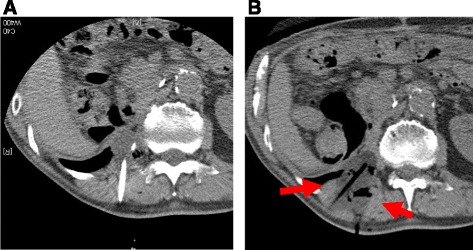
a, b. Multiple synergistic probes were inserted into the tumor to ensure complete encapsulation of the lesion by the ice ball. The ice ball was periodically monitored using CT to secure at least a 1-cm margin around the tumor
